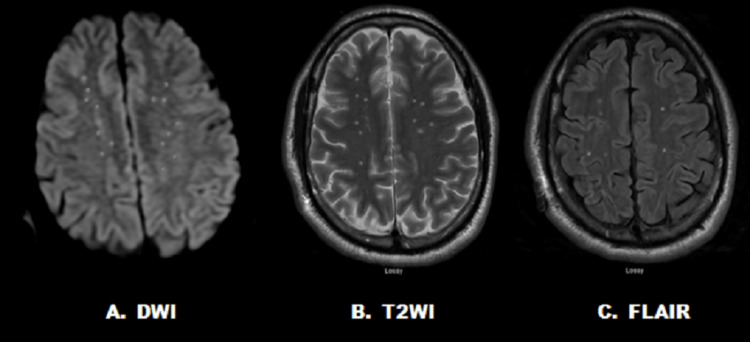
Case 1 with numerous cerebral white matter hyperintensities on (A) DWI, (B) T2WI, and (C) FLAIR MRI sequences within five days of traumatic injury DWI, diffusion-weighted image; T2WI, T2-weighted image; FLAIR, fluid-attenuated inversion recovery; MRI, magnetic resonance imagining.
